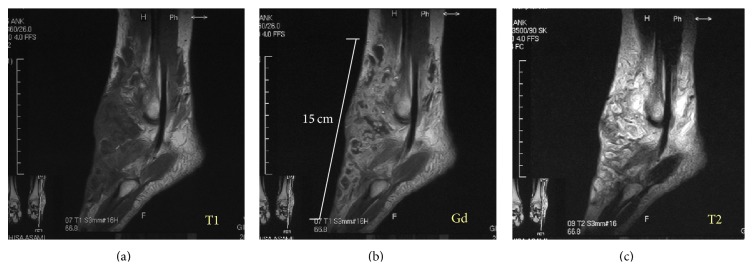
MRI showing an ill-defined heterogeneous soft tissue mass. T1 weighted image (a) shows the lobular low signal area in ill-defined border from iso- to low signal lesion, where it is enhanced by gadolinium (b) on the anterolateral part of the ankle. T2 weighted image (c) shows ill-defined delineated mixed high signal and low signal lesion.
